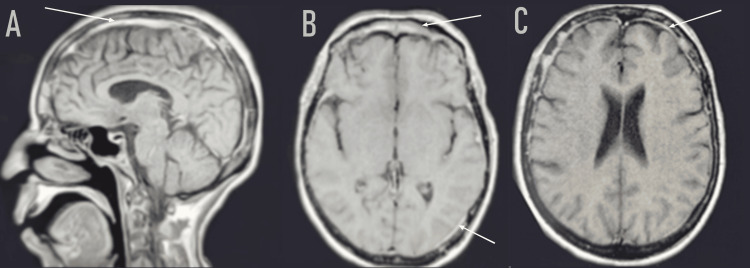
Brain MRI with T1-weighted sequence post-gadolinium injection, sagittal (A) and axial (B, C) sections revealing diffuse meningeal thickening (white arrows)
